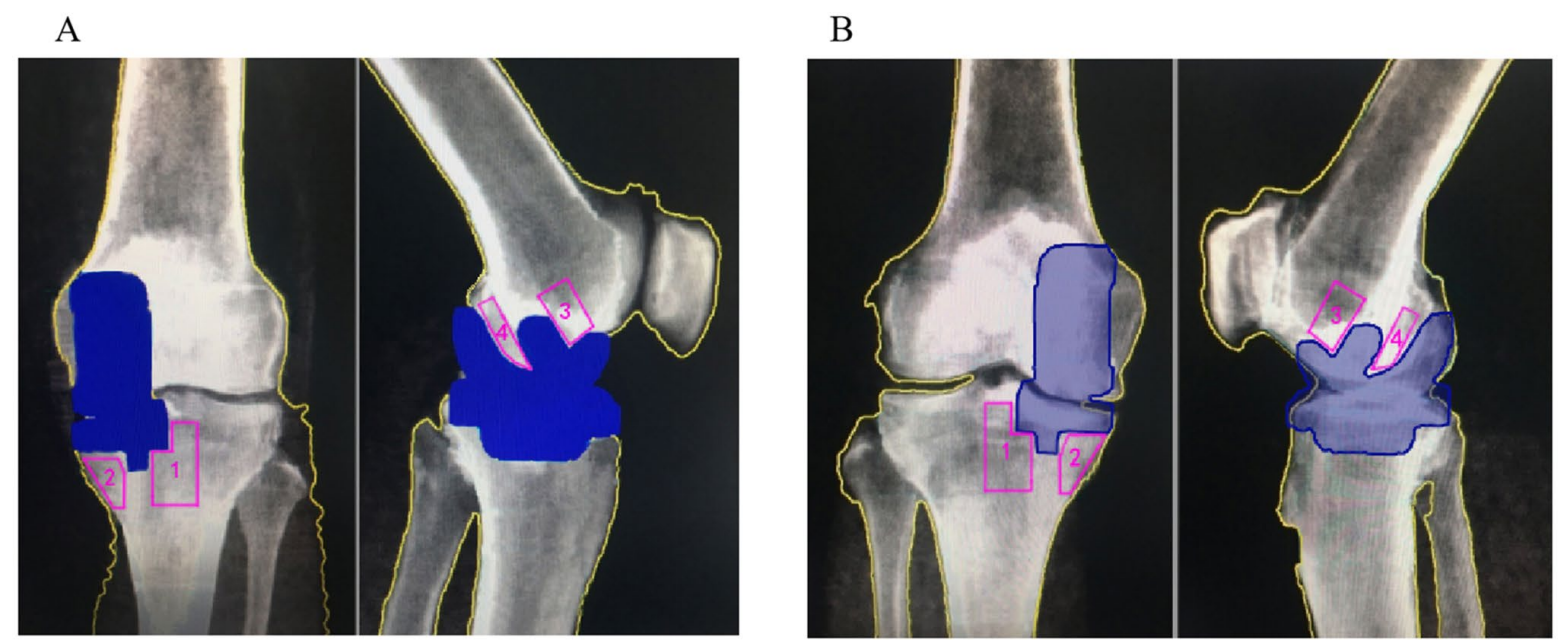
The location of ROI sinantero-posterior DEXA scans. A: Operated knee. B: Unoperated control knee. A diaphysial midline was used to divide the medial (ROI1) and lateral (ROI2) ROIs. ROI 1 was the lateral side of the tibial prosthesis keel, and ROI 2 was the medial side of the tibial prosthesis keel. The ROI around the femoral prosthesis was divided using lateral knee radiography and was also divided into two ROIs: ROI 3 before the femoral prosthesis column and ROI 4 after the femoral prosthesis column
